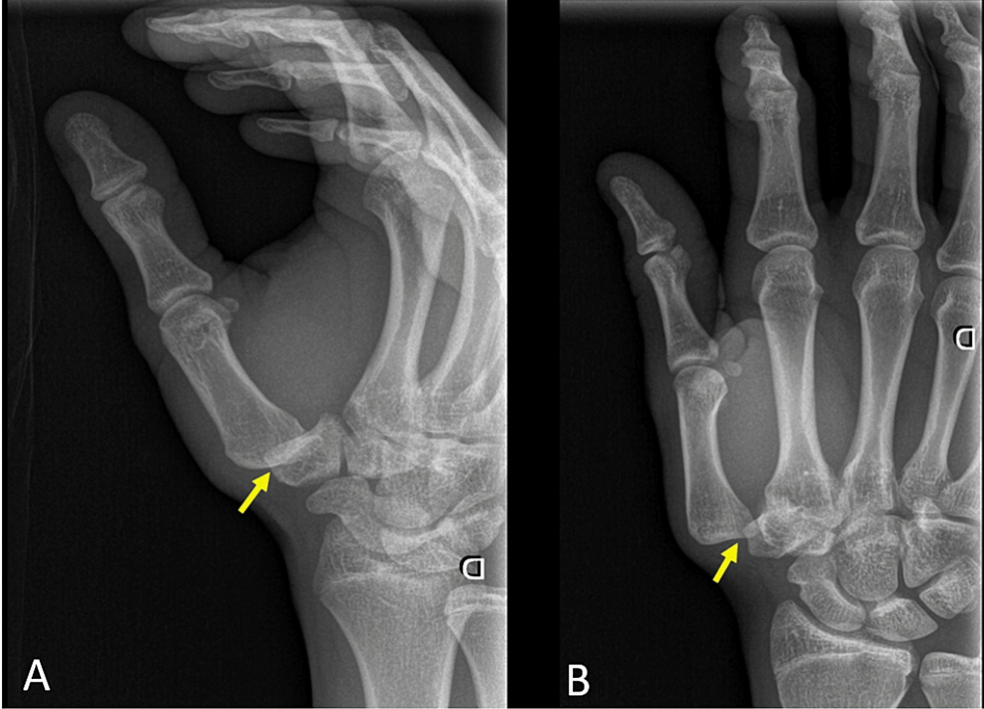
(A, B) Anteroposterior and oblique radiographs of the right hand showing isolated dislocation of the right TMJ without any associated fractures.
